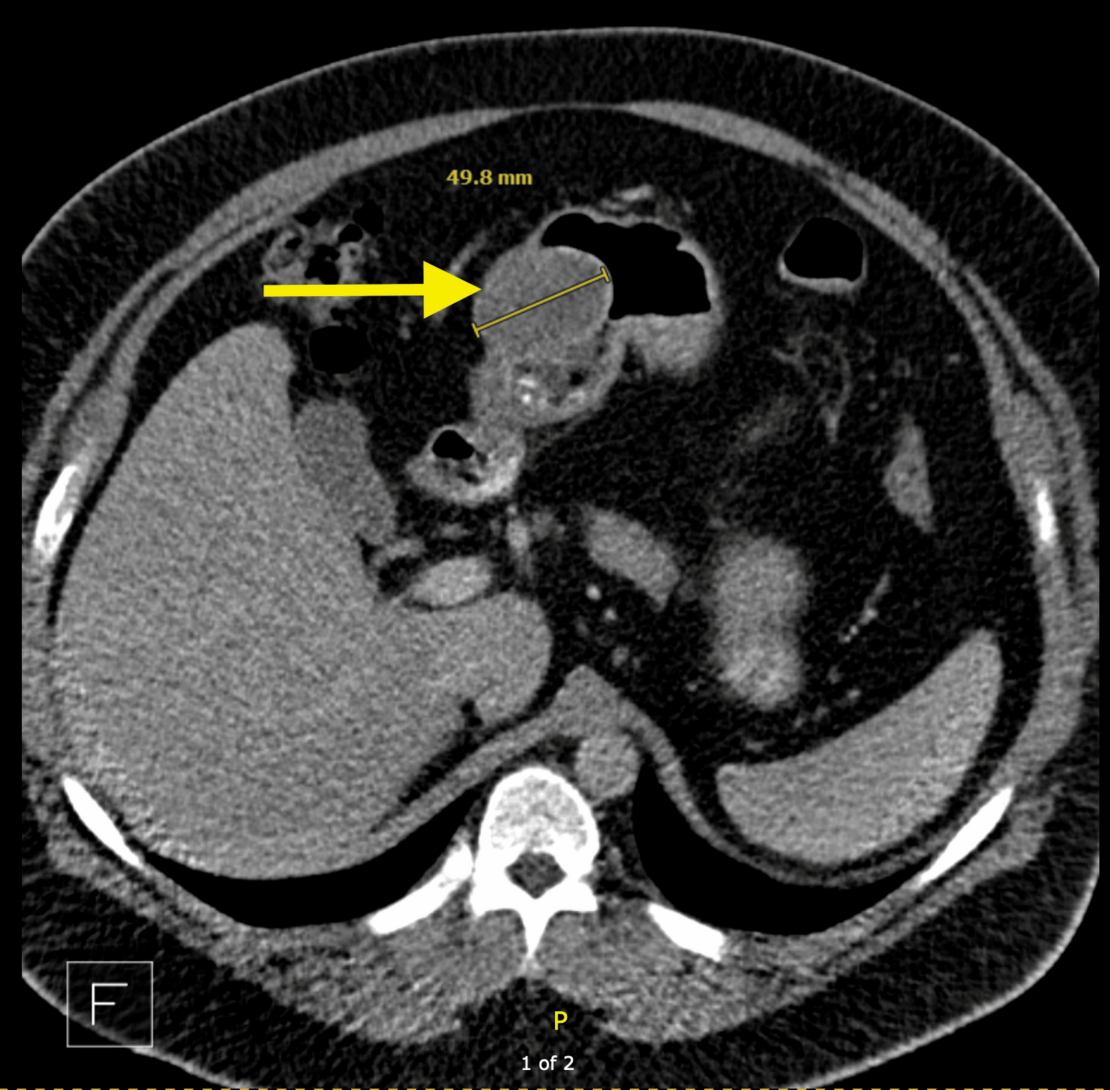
CT showing a likely submucosal mass (arrow) with smooth margins, involving the anterior gastric antrum
